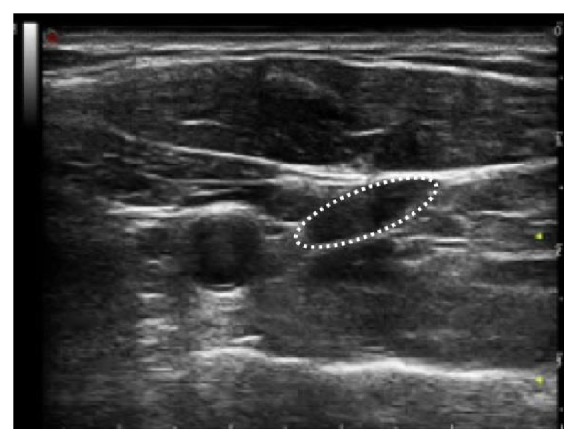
High resolution B-mode ultrasound imaging of the same case, in the transversal access of the neck, shows the elliptical shape of the internal jugular vein, outlined by the interrupted line.
